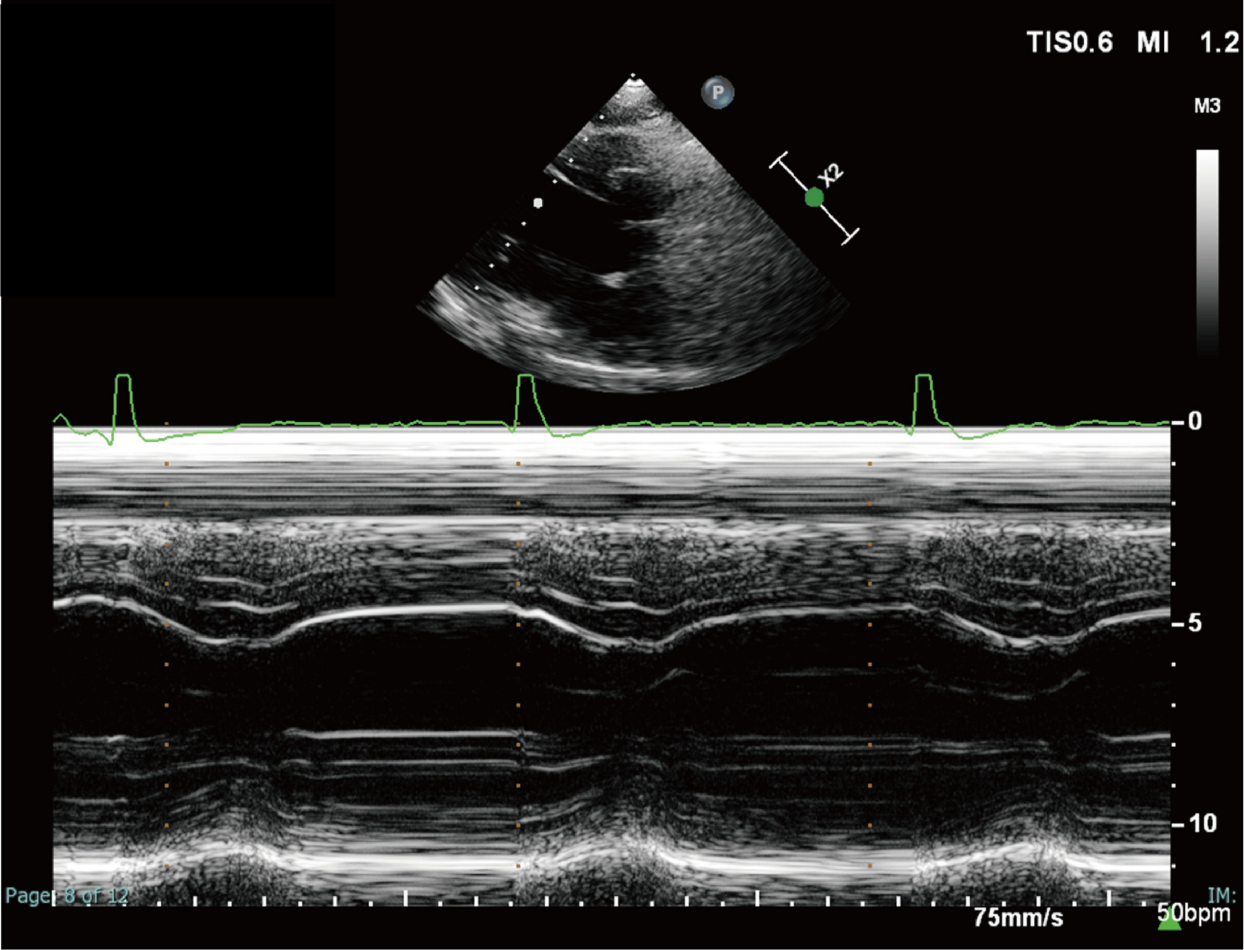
Preoperative echocardiogram M-mode echocardiography shows a long-axis cross-sectional image of the ventricle at the level of the tendon cords. The ultrasound probe was placed on the left margin of the fourth sternal rib. Left ventricular end-diastolic and -systolic diameter was 50 and 33 mm, respectively. Left ventricular ejection fraction and right ventricular area change were 63% and 35%, respectively. All of these measurements were within normal limits.
